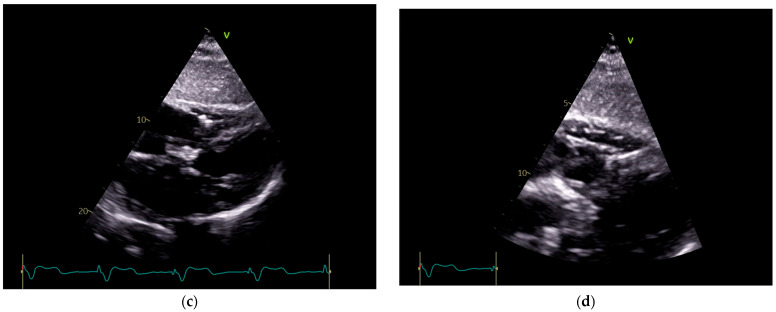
Echocardiography exam images at admission: A transthoracic echocardiography (TTE) exam was performed in the apical 4-chamber view (a,b) and subcostal view (c,d), revealing the insertion of the pacing probe into the myocardium of the right ventricle free wall, without any visible fractures or ruptures of the lead.
